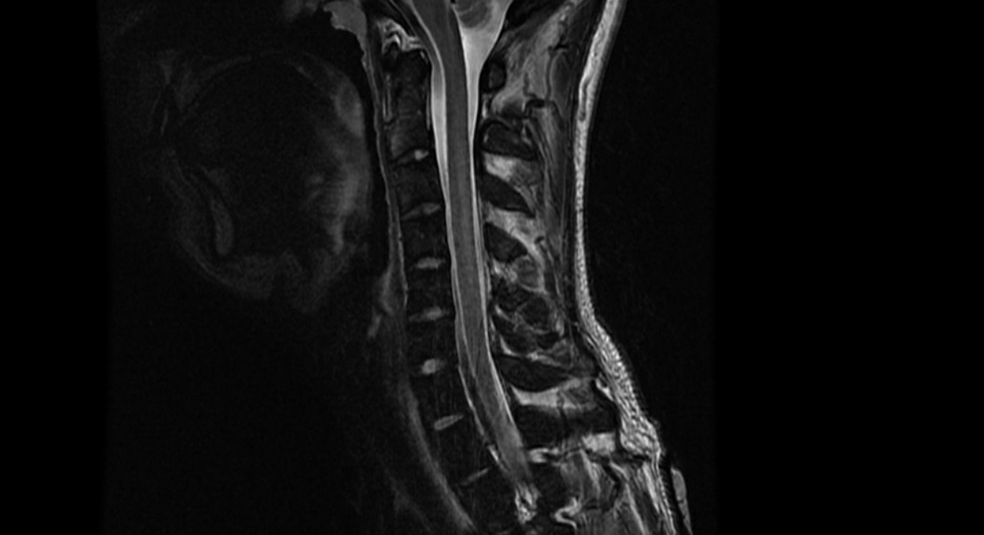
MRI T2-weighted sagittal view of the cervical spine showing increased T2 signal within the dorsal aspect of the cervical spinal cord from C2 to C5
